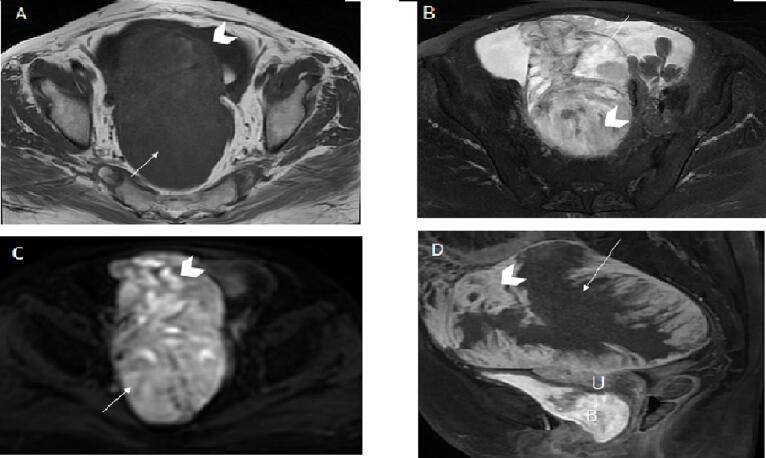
The cystic component (arrow) shows slight hyperintensity on axial T1WI (A), homogeneous hyperintensity on axial T2WI with fat suppression (B), no enhancement on contrast-enhanced T1WI with fat suppression (C), isointensity on DWI (D). The mural nodule (arrow head) demonstrates isointensity (A), heterogenous hyperintensity (B), marked enhancement (C) and hyperintensity on DWI (D). U: uterus, B: bladder.
